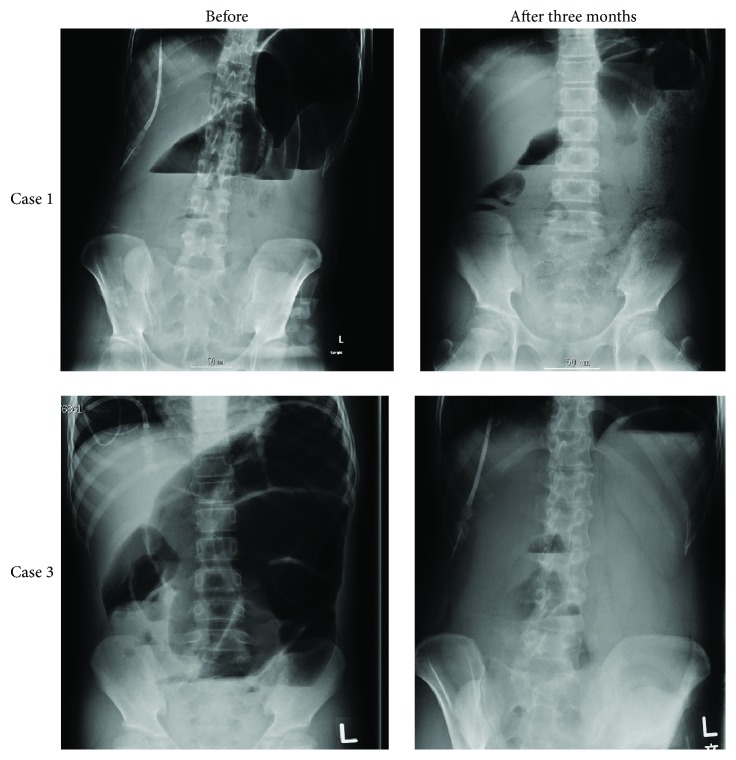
Comparison of abdominal roentgenograms in Cases 1 and 3 obtained before and three months after the oral administration of NJT. Improvements in intestinal dilatation and niveau formation were observed at three months after the oral administration of NJT.
